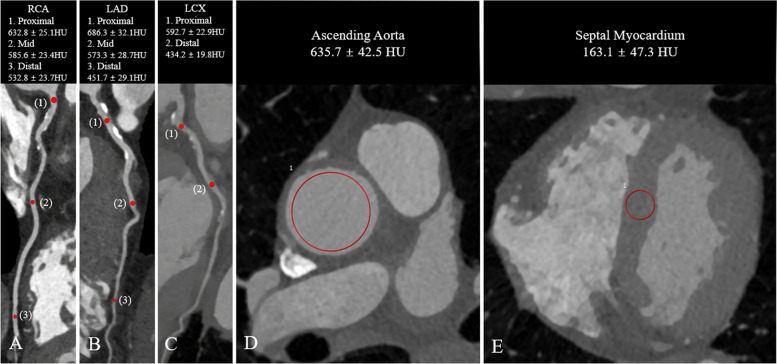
Quantitative assessment of coronary computed tomography angiography (CCTA) image quality. A–C Curved multiplanar reformations of the right coronary artery (RCA), left anterior descending artery (LAD), and left circumflex artery (LCX), showing regions of interest (ROIs) placed in the proximal and distal coronary segments for attenuation measurement. D Ascending aortic ROI used for image noise measurement. E Septal myocardial ROI used for myocardial attenuation measurement. HU, Hounsfield units
